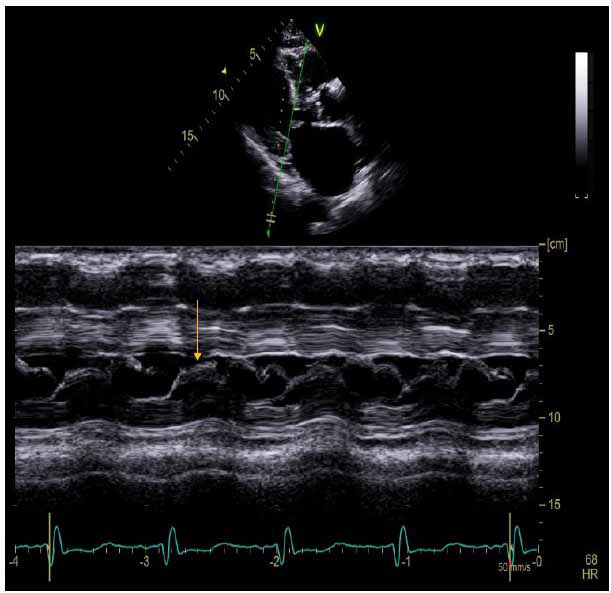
M-mode echocardiographic tracing of the mitral valve in a middle-aged patient with HOCM, revealing the systolic anterior movement of the anterior mitral leaflet (yellow arrow).
